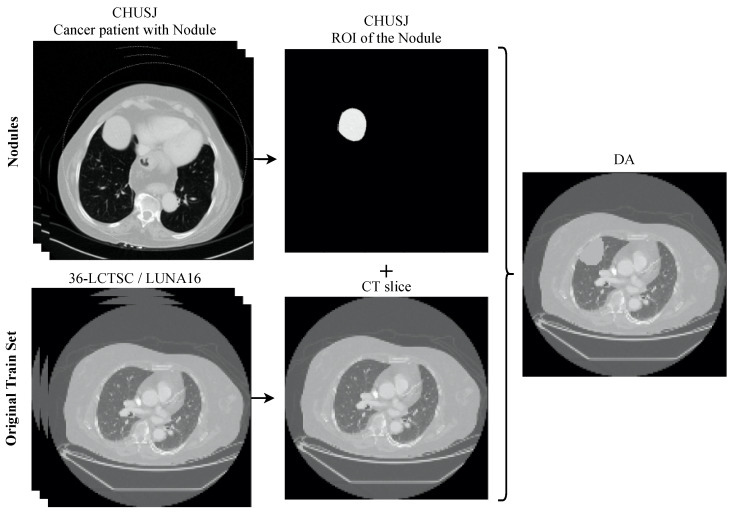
Overview of the process to generate the data augmentation. The nodules from the dataset CHUSJ were added to the original training set (36-LCTSC and LUNA16), creating new CT slices with multiple pathological imaging findings.
